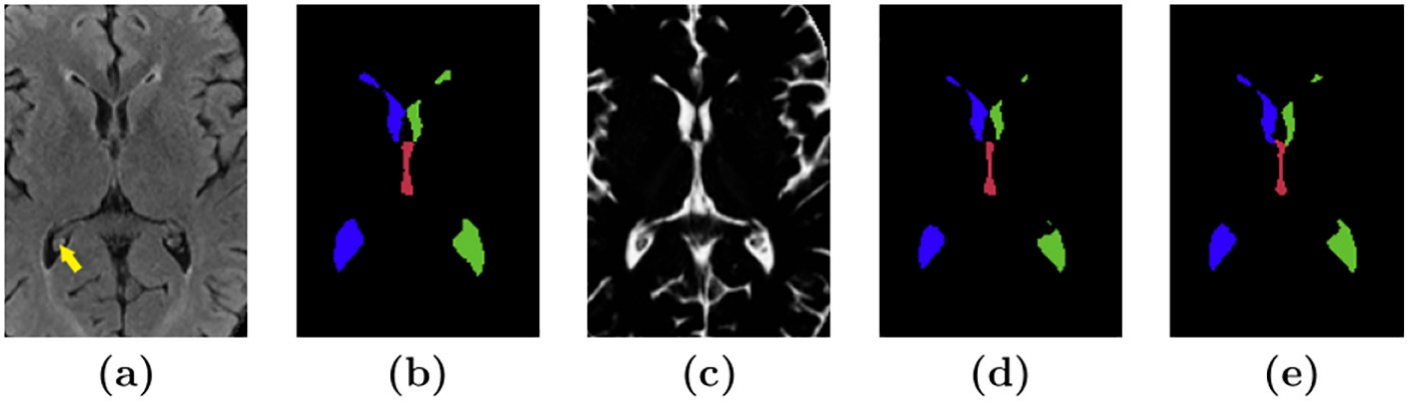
Preparation of training labels. Image (a) shows a slice of a FLAIR image showing choroid plexus in the right lateral ventricle (yellow arrow). Image (b) shows the corresponding ventricle segmentation from RUDOLPH, and (c) shows the corresponding CSF segmentation from SegAE. Image (d) shows a ventricle segmentation obtained by element-wise multiplication of each label from RUDOLPH with the CSF segmentation in (c) and a morphological closing of the lateral and third ventricles. (e) shows a corresponding manual delineation.
